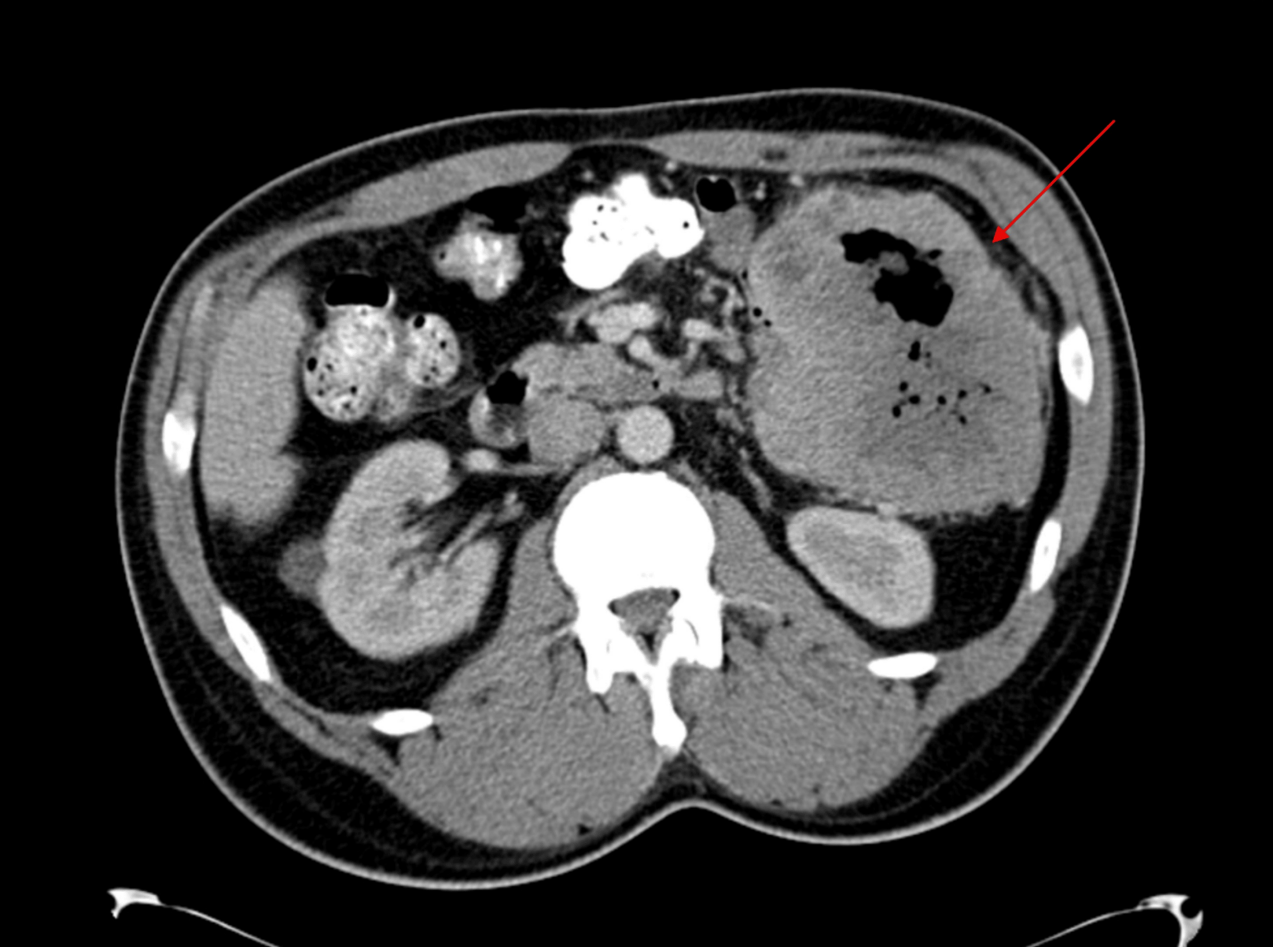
Axial view of contrast-enhanced CT of the abdomen and pelvis showing a large left-sided abdominal mass measuring 11.7 x 11.7 x 10.3 cm (red arrow), closely associated with the small bowel and descending colon.
